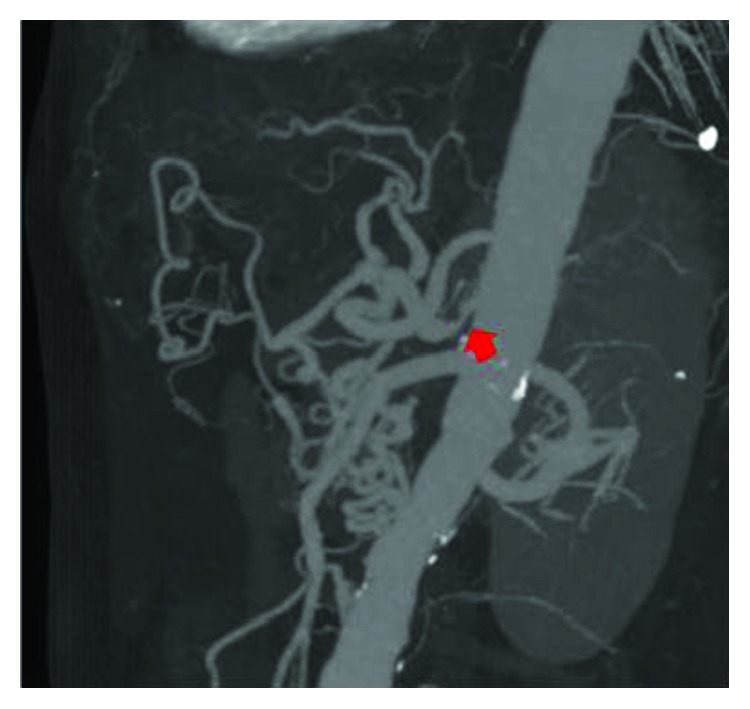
Case 2: the CT scan shows stricture of a root of the celiac artery by MAL (arrow).
